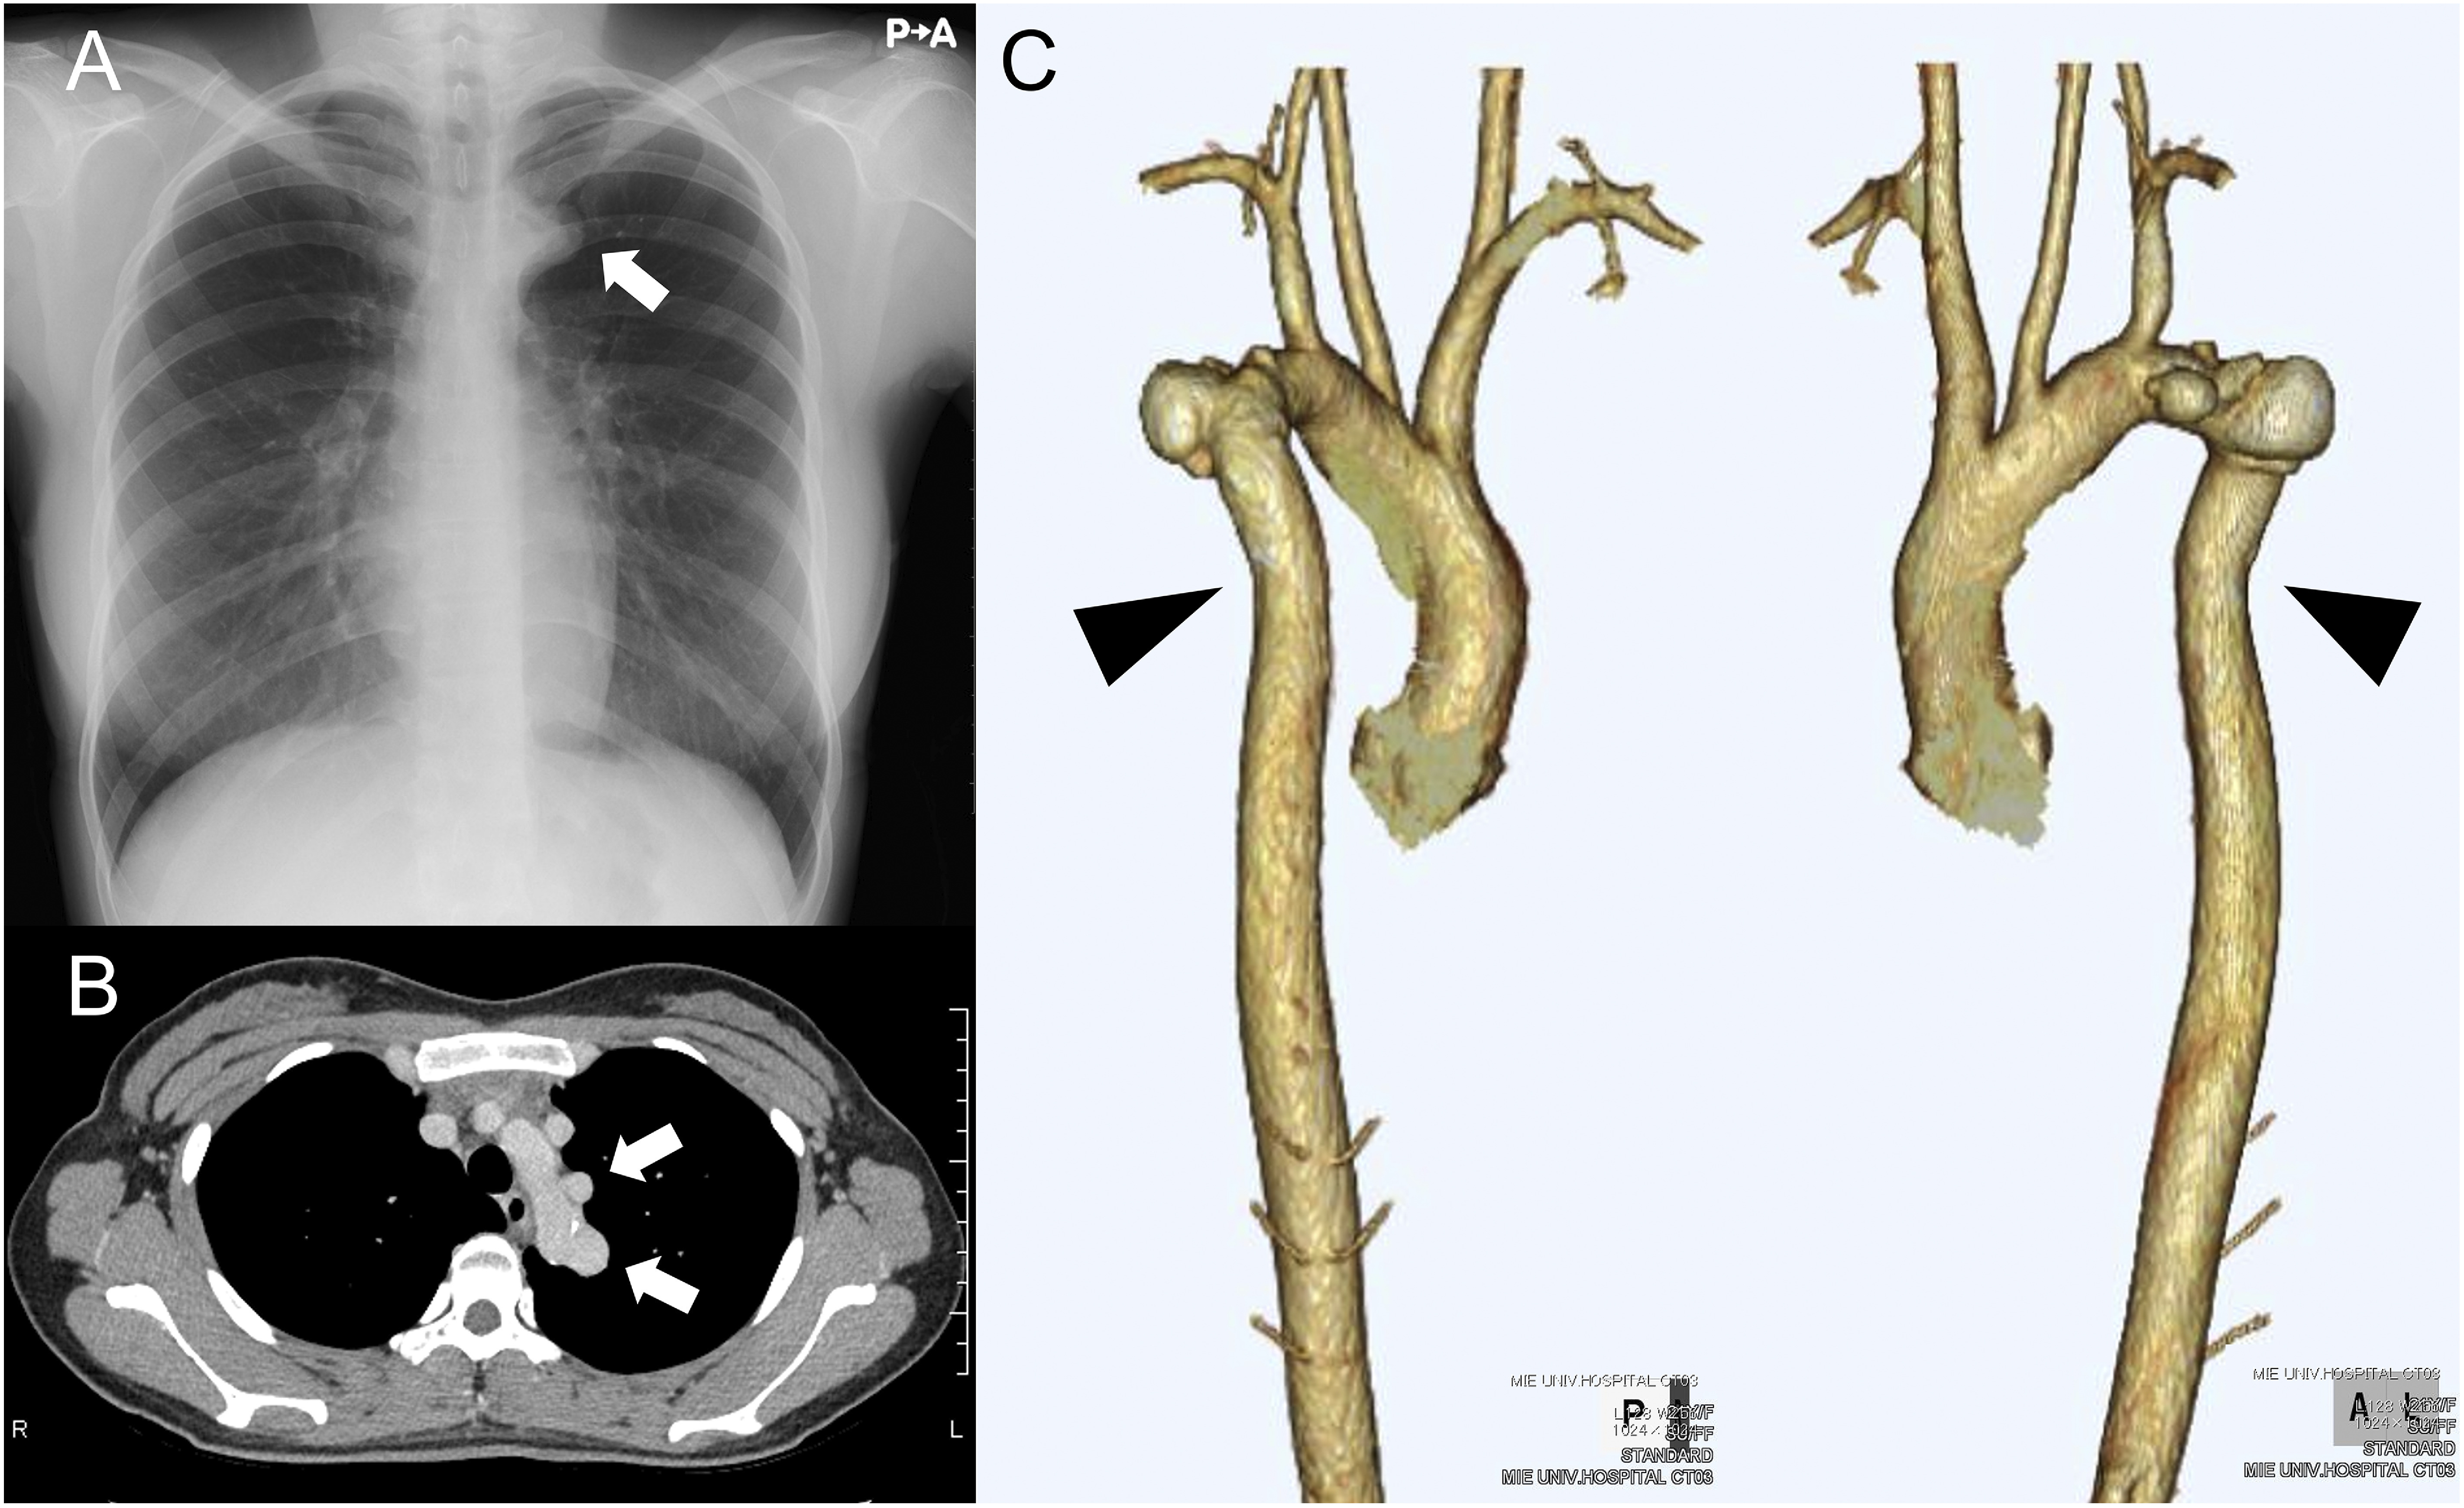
Fig. 1 (A) Chest X-ray image. The white arrow indicates the aneurysmal shadow of the aorta. (B) Cross-sectional computed tomography (CT) image of the saccular aneurysms (white arrows). (C) Three-dimensional CT angiographic images demonstrating the saccular aneurysms and kinking of the descending aorta (black arrowheads).
